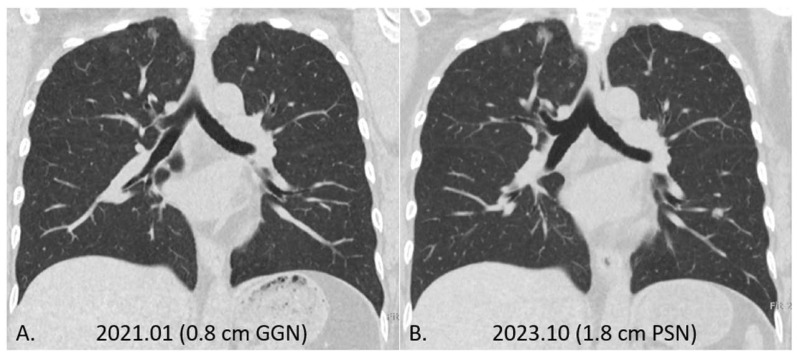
A 50-year-old woman with a family history of lung cancer underwent a baseline CT scan of the lungs in January 2021, which identified a 0.8 cm pure ground-glass nodule in the right upper lobe (A). An active surveillance approach was selected for follow-up. Over the next two years and nine months, the nodule exhibited interval growth, increasing to 1.8 cm (B). Surgical resection later confirmed the diagnosis of invasive lung adenocarcinoma. This case underscores the critical need for careful monitoring and comprehensive assessment of subsolid nodules, particularly in non-smokers with a familial predisposition to lung cancer, who face an elevated risk of progression to invasive malignancy. Identifying high-risk lesions necessitates a multifaceted evaluation incorporating clinical, imaging, and genetic factors.
